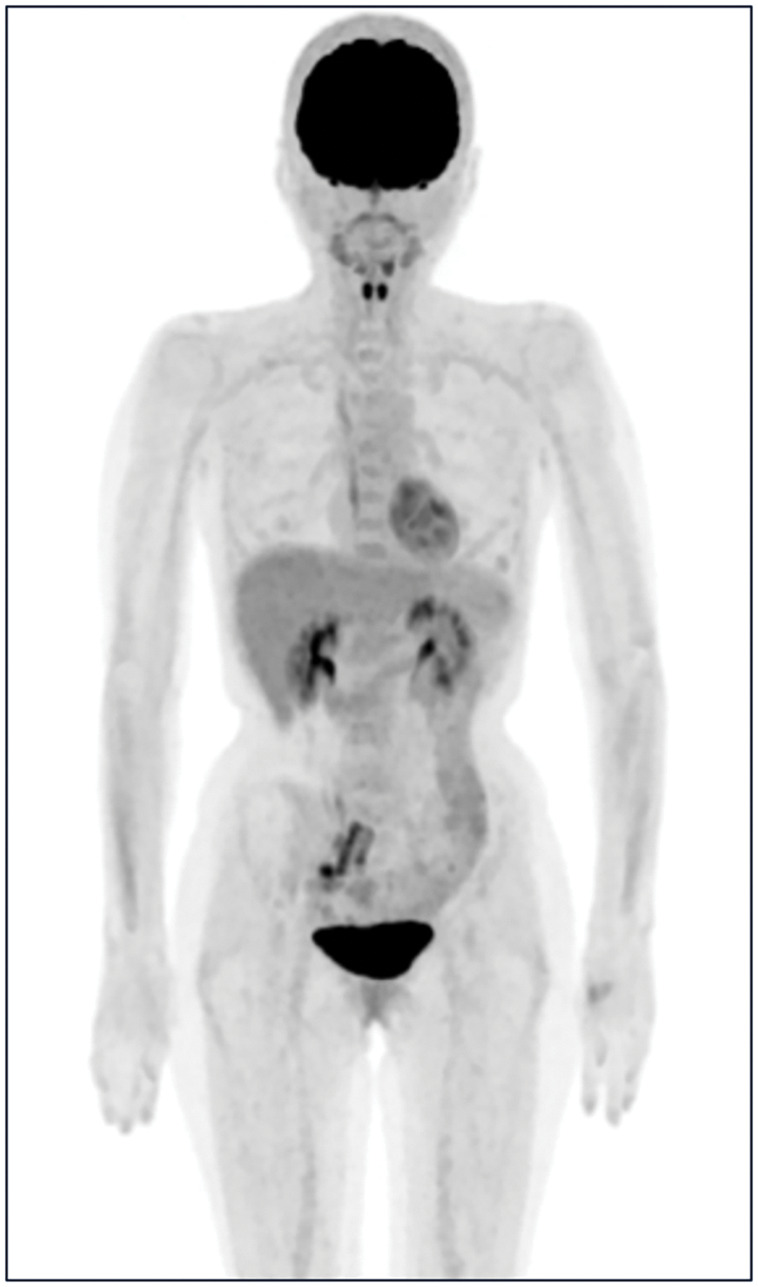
PET-CT findings. The results of the PET-CT performed after referral to our department are shown.
